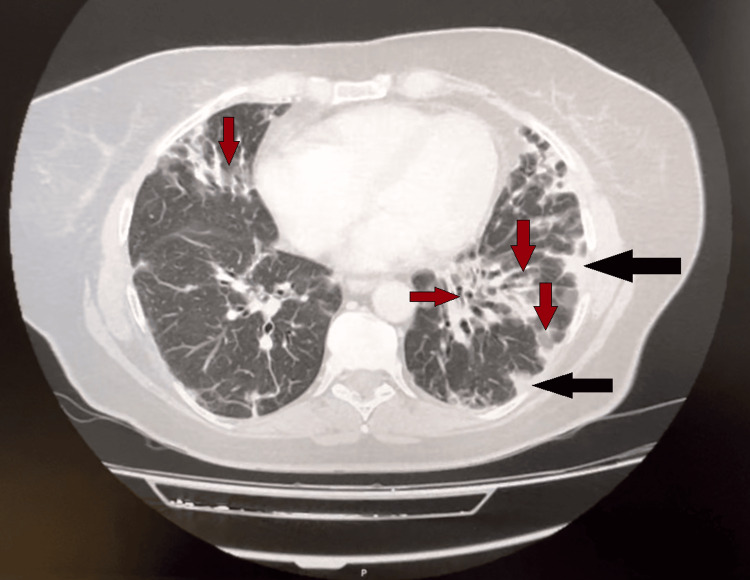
Axial view of the lungs from a CT scan of the chest, abdomen, and pelvis CT: Computed Tomography The black arrows show subpleural consolidations in the left lung, and the red arrows show ground-glass opacities and bronchiectasis in the right and left lungs.
